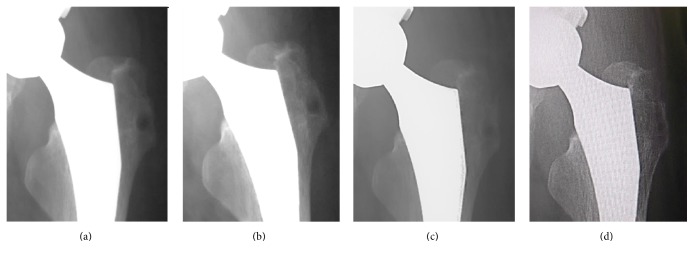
Radiographs of the left hip joint after the removal of the Ethibond suture and the granulation tissue. Bone tunnels gradually decrease and disappear. There is no evidence of osteolysis of the greater trochanter at 10 months after the second surgery. (a) At 1 month after the second surgery. (b) At 2 months. (c) At 3 months. (d) At 10 months.
